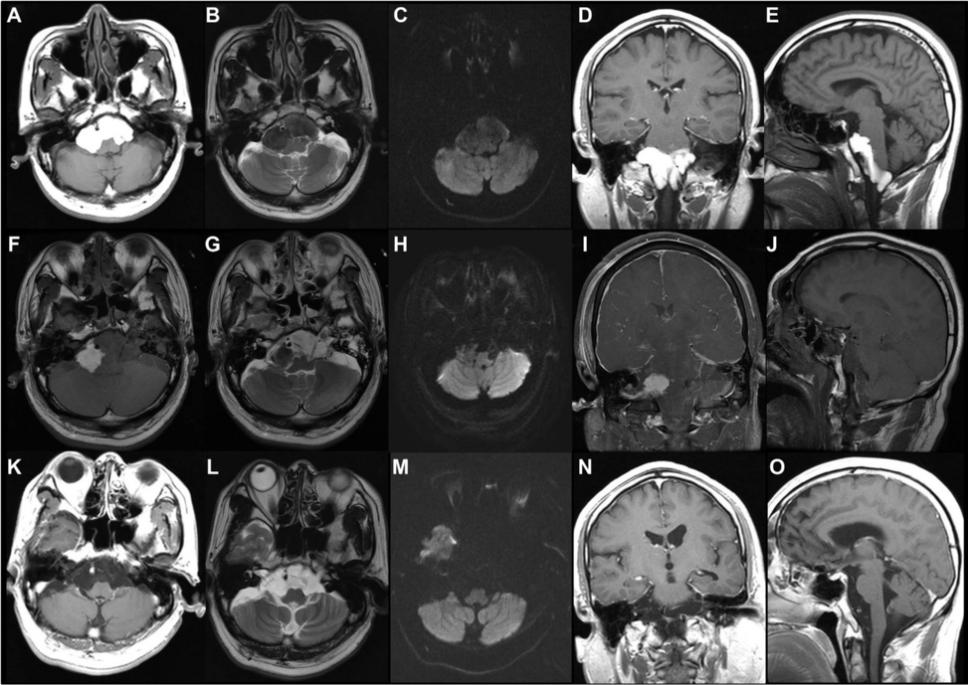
Preoperative and postoperative MR imaging. In the preoperative MR image, a well-defined, lobulated mass with a size of about 5.6 × 2.7 × 6.1 cm is seen in front of the pons to the medulla with several internal septae. The mass showed hyper-intensity on T1-weighted, hypo-intensity on T2-weighted, and hypo-intensity on diffusion-weighted images. The superior portion of this cystic lesion compresses the lower portion of the pons. The inferior portion of the lesion extends posteriorly and is surrounding the medulla, extending to the C1-C2 intervertebral level (a–e). Postoperative MR imaging after a grossly subtotal surgical resection was done through the minimal lateral supraorbital approach (f–j). In the 15-month follow-up MR images, the remnant cyst mass completely disappeared (k–o)
